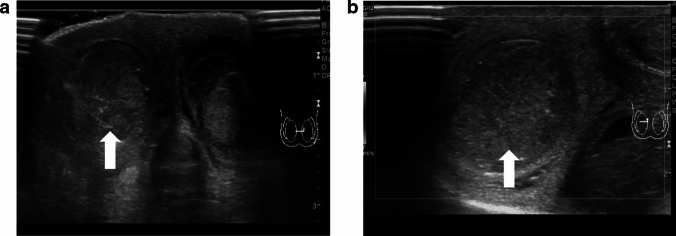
Ultrasonographic images of a 0-day-old neonate with right testicular torsion. a Swelling of the right testis is evident. b Heterogeneous echogenicity is observed within the affected testis. Testicular torsion and necrosis due to torsion were surgically diagnosed
